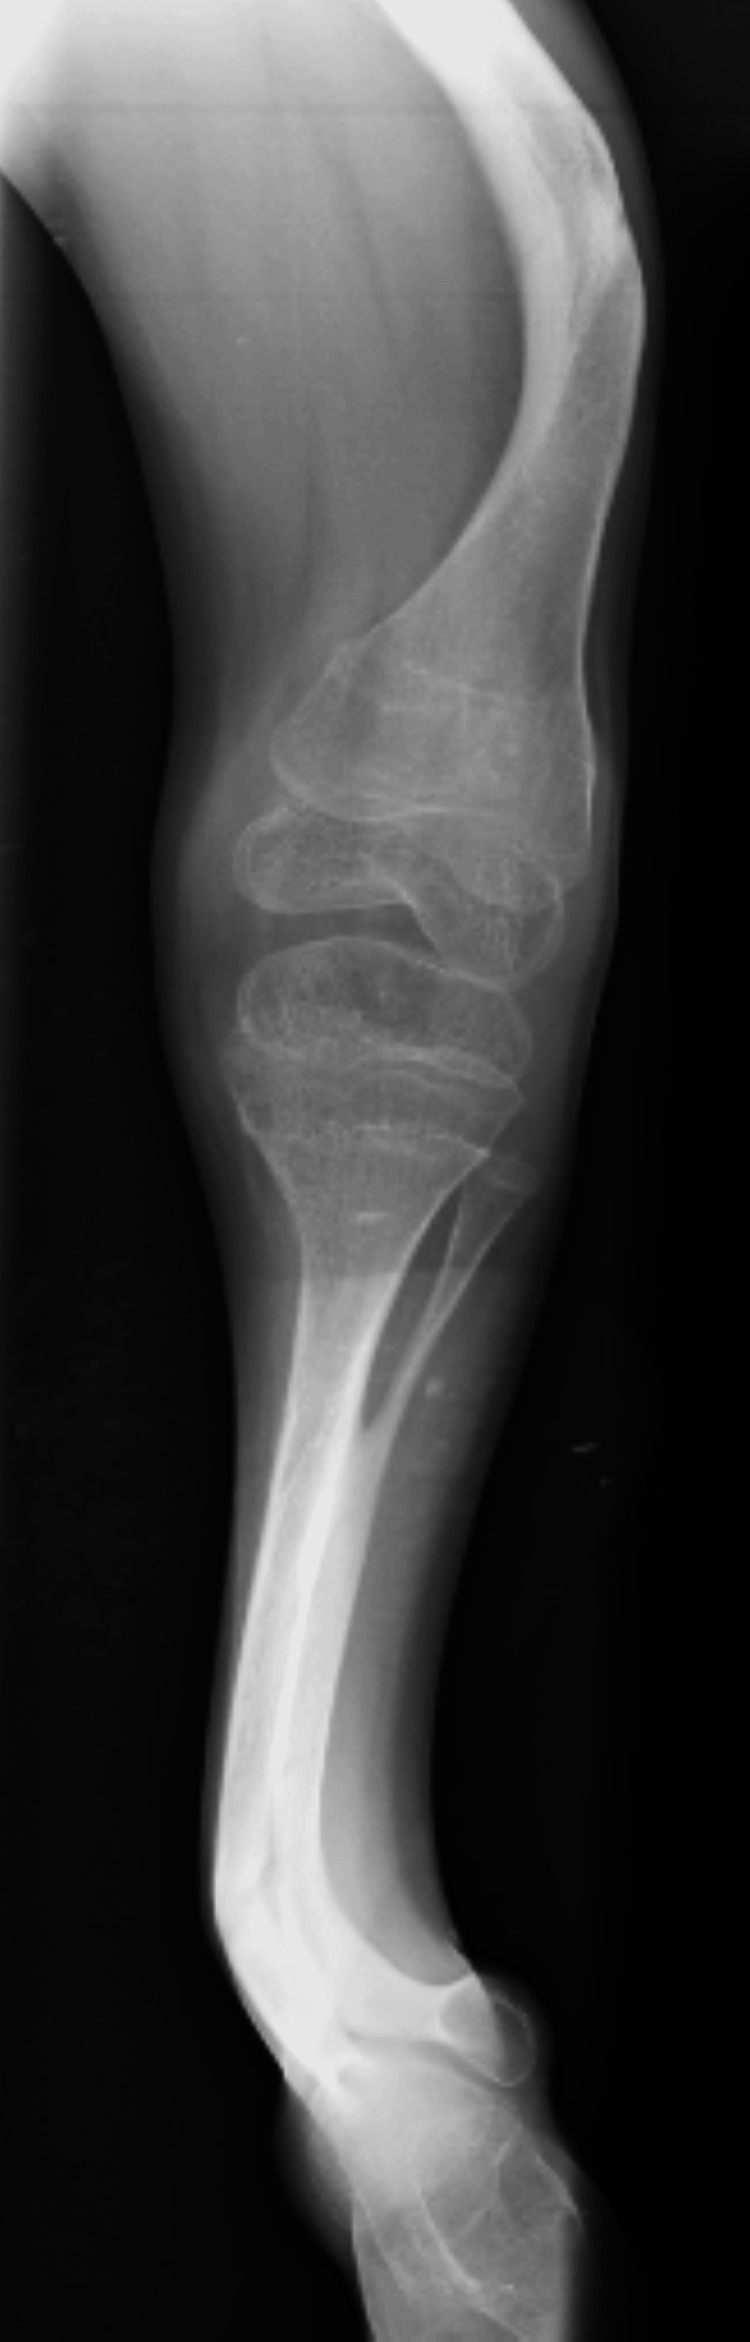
X-ray of the right tibia and fibula.
